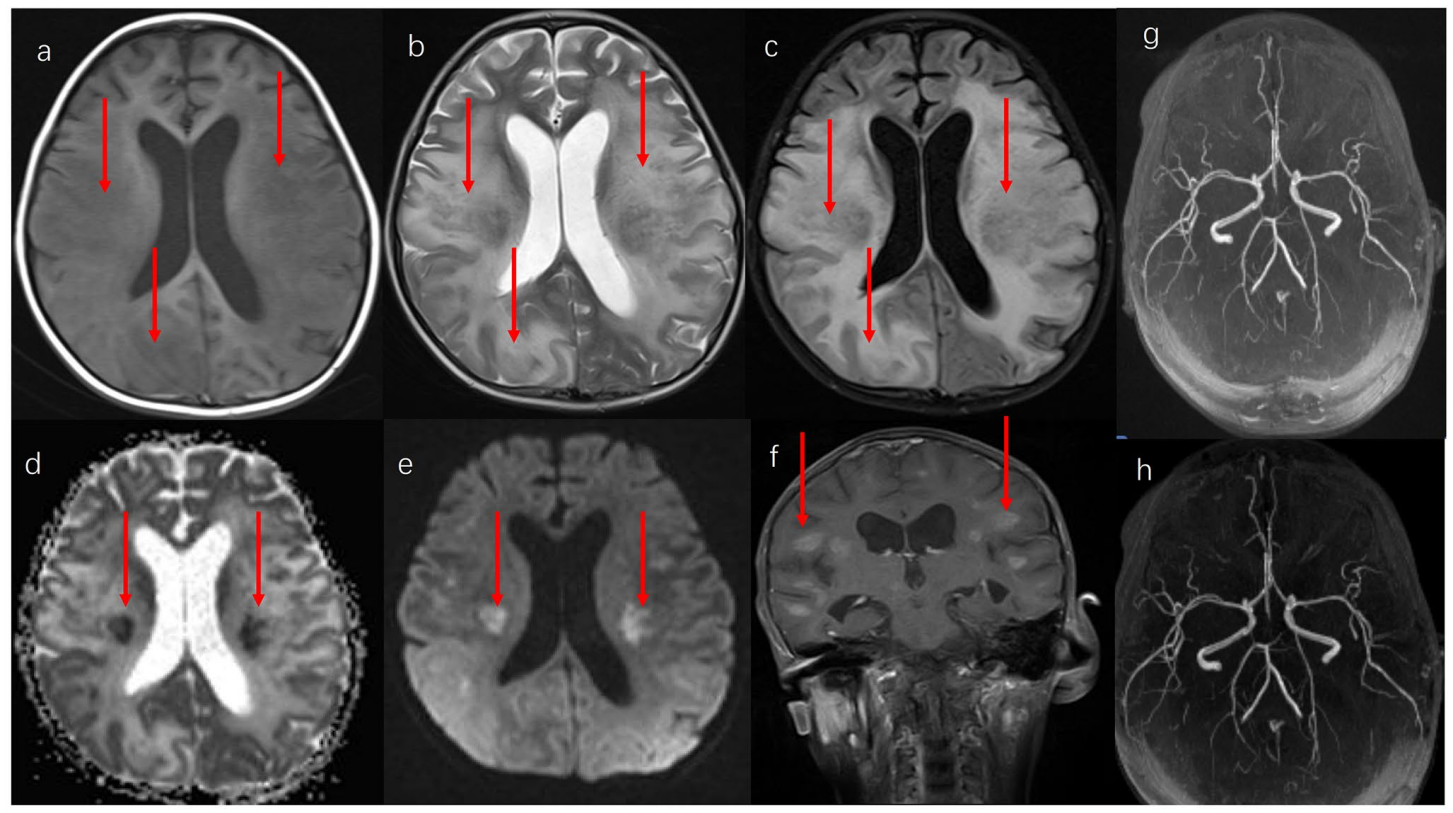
Brain MRI revealed multiple lesions in the bilateral cerebellar hemispheres, thalamus, basal ganglia region, lateral ventricle, radial coronal, centrum semiovale, bilateral frontoparietal temporal lobe, and right occipital lobe. a: T1 weighted imaging (T1W1) revealed slightly low signal. b: T2 weighted imaging (T2WI) showed high signal. c: Flair image presented a slightly high signal intensity with blurred boundaries. d and e: Diffusion-weighted imaging (DWI) suggested limited diffusion of some lesions. f: Enhanced MTI suggested mild enhancement of some white matter lesions. g and h: There was no obvious abnormality on the brain vessel MRI
